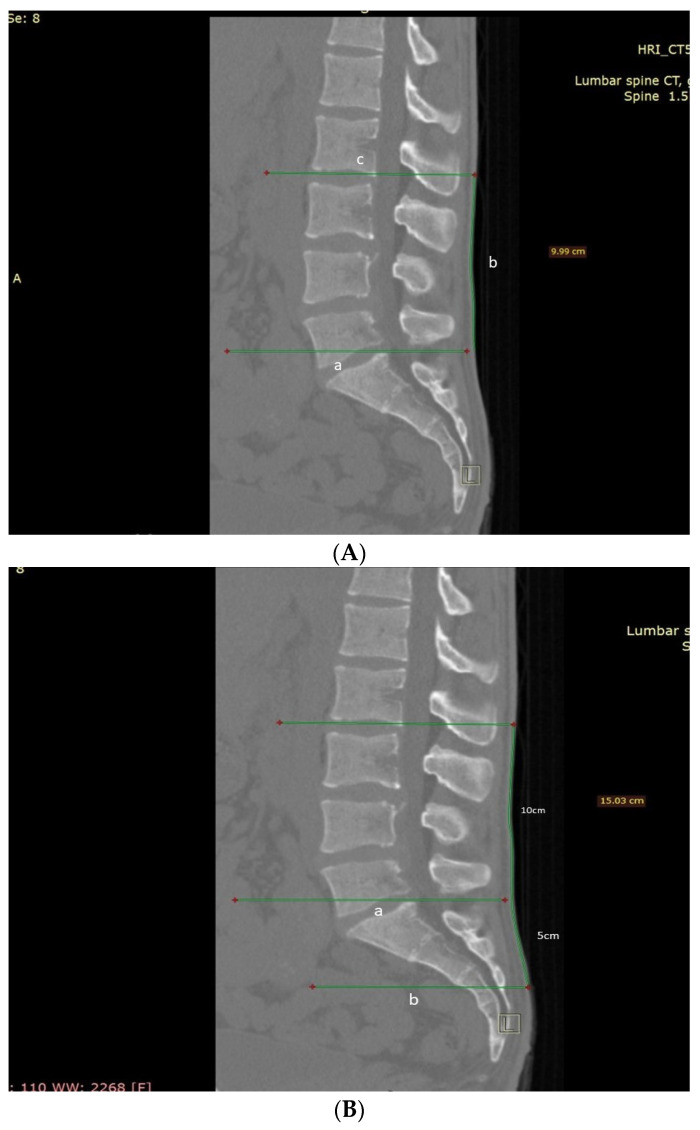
(A) Schober Test CT measurement. A parallel line is drawn from the posterosuperior corner of the S1 vertebra to the skin (marked as a), which is followed by a 10 cm line drawn over the skin to reach the cephalad endpoint imitating the original Schober test (marked as b). A parallel line was drawn from this point to the skeletal spine level, transecting a vertebra (upper third, middle third, or lower third) or a disc space (marked as c). Lumbar vertebrae were numbered as (L)1 to (L)5, with the upper third as 0.3, middle third as 0.6, and lower third as 0.9. A line transecting the middle third of L2 was therefore designated as 2.6. Sacral vertebras were numbered from 6 to 10 similarly. (B) Modified Schober Test CT measurement. It was measured similarly to the Schober test by marking a skin point 5 cm caudal and 10 cm cephalad to the lumbosacral junction (a parallel line drawn from the posterosuperior corner of the S1 vertebra to the skin) (Marked as a). Spinal endpoints were measured; the cephalad point was measured as in the Schober test. The caudal end was measured as the parallel line from the caudal 5 cm skin point to the skeletal spine level (upper third, middle third, or lower third of a vertebra or the disc space) (Marked as b).
